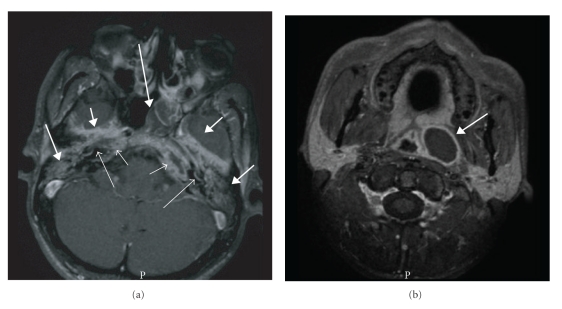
(Case A) Axial T1-weighted MR image with fat suppression after administration of Gadolinium at the level of the petrous bones (a) and just below the skull base (b). Indicated in a are the bilateral inflammatory changes in the petrous apices (short open arrows), bilateral involvement of the basal turn of the cochlea (long open arrows), extensive bilateral pachymeningeal involvement at the temporal lobes (short closed arrows), opacification of both mastoids and middle ears (medium closed arrows), and the mass in the left middle nasal passage extending up to the left Eustachian tube as visualized during fiber endoscopy (long closed arrow). Extension of the abscess into the parapharyngeal space, resulting in a bulge of the contour of the nasopharynx, is also shown (b, medium closed arrow).
